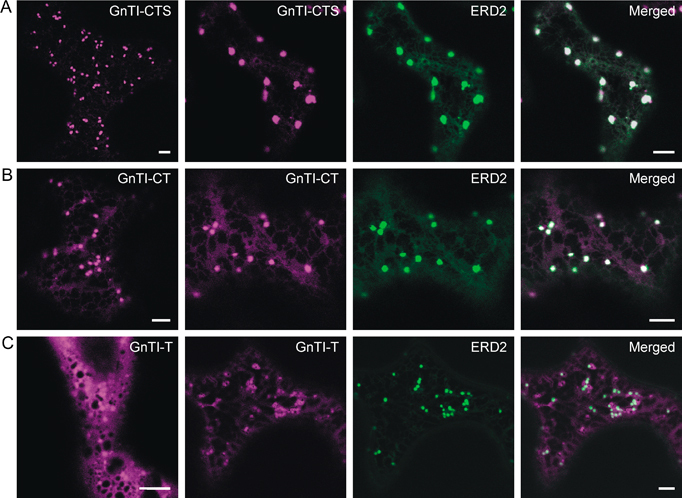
The correct targeting of GnTI to its steady-state location relies on signals located in the cytoplasmic tail and transmembrane domain.A) Confocal images of *N. benthamiana*leaf epidermal cells expressing GnTI-CTS-mRFP either alone or together with ERD2-GFP. The GnTI-CTS region targets mRFP predominantly to the Golgi. B) When expressed alone or coexpressed with ERD2-GFP, GnTI-CT-mRFP accumulates mainly in the Golgi, demonstrating that the luminal stem region is not needed for GnTI localization. C) In contrast, GnTI-T-mRFP highlights the cytoplasm and punctae of mostly unknown nature that only partially colocalize with ERD2-GFP. Scale bar = 5 μm for all images.
